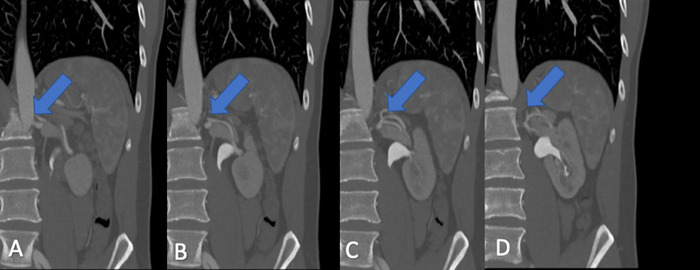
Coronal computed tomography angiography images of the donor's left kidney demonstrate complex extrarenal bifurcation and crossing of 2 main arteries, highlighting the ambiguity regarding the number of arteries potentially present after donor nephrectomy. Arrows point to the arteries. From left to right (A to D), the images become more posterior.
